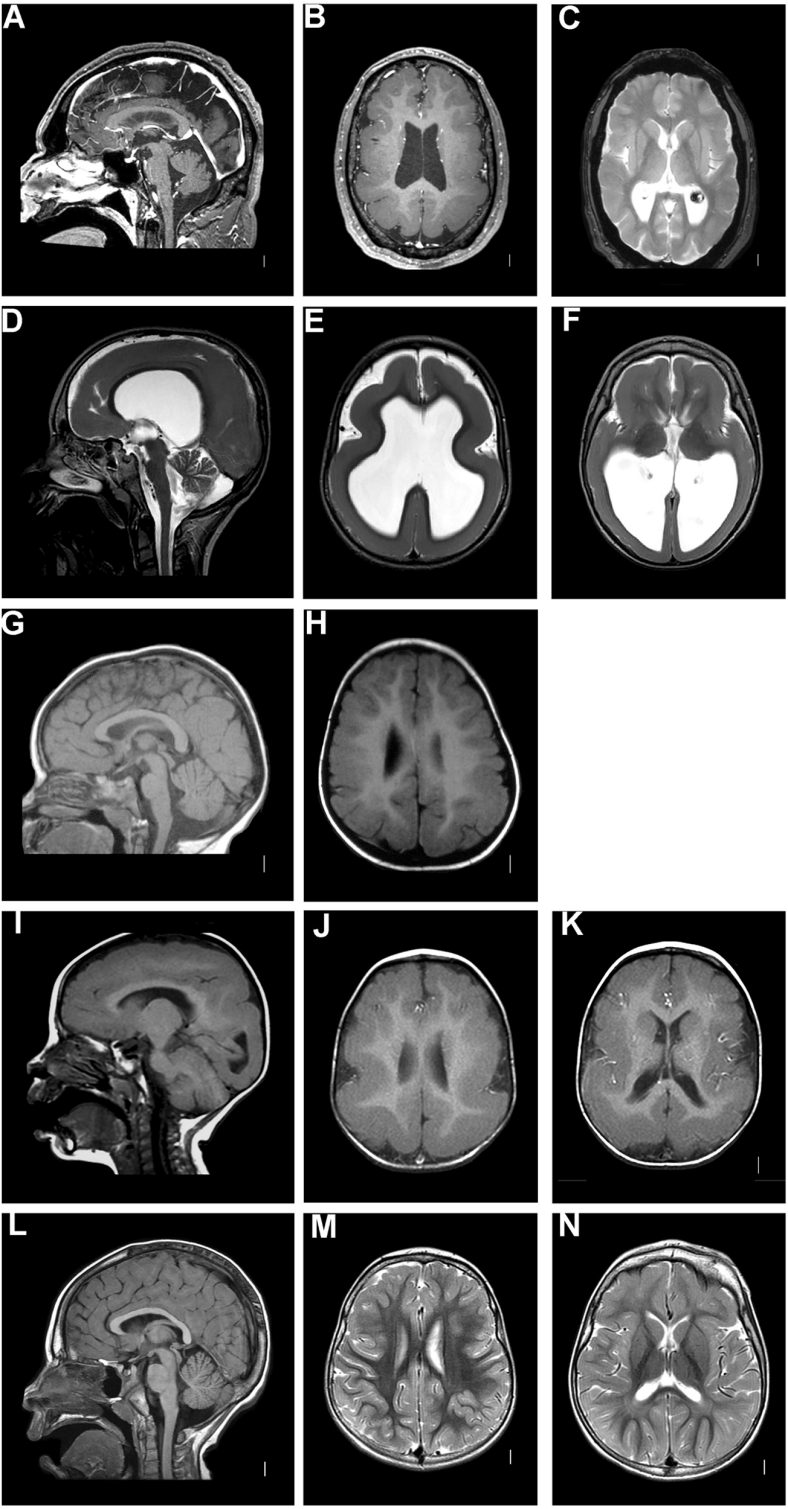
Imaging characteristics patients 1–4 and a normal subject. Brain MRI of patient 1 at age 36 years. Sagittal planes through the midline (a) show a normal corpus callosum, brainstem, and cerebellum. Axial T1-weighed images (b) and T2-weighed images (c) show pachygyria with a posterior to anterior gradient, enlarged posterior horns of the lateral ventricles, and enlarged perivascular spaces. Brain MRI of patient 2 at age 11 years. Sagittal planes (d) show a thin corpus callosum, hypoplasia of the brainstem, and the cerebellar vermis. Axial T2-weighed images (e, f) show diffuse lissencephaly, reduced white matter, and enlarged lateral ventricles and dysplastic basal ganglia. Brain MRI of patient 3 at age 1 year 6 months (g, h) and patient 4 at age 12 months (i, j, k). Sagittal planes (g, i) show a normal appearance of corpus callosum, brainstem, and the cerebellum. Axial T1-weighed images (h, j, k) show pachygyria with a posterior to anterior gradient, enlarged lateral ventricles, and reduced white matter. Brain MRI of a healthy subject (age 4 years). Sagittal image (l). Axial T2-weighed images (m, n)
